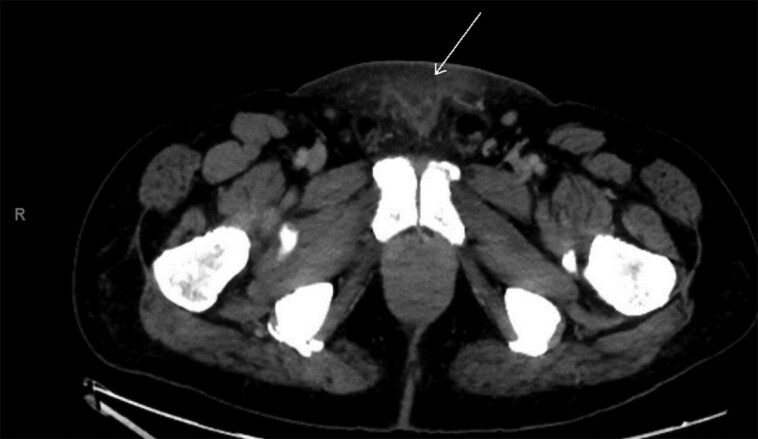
Computed tomography image of abdomen, demonstrating a marked fat stranding with skin induration of anterior suprapubic subcutaneous tissue compatible with cellulitis (arrow).
